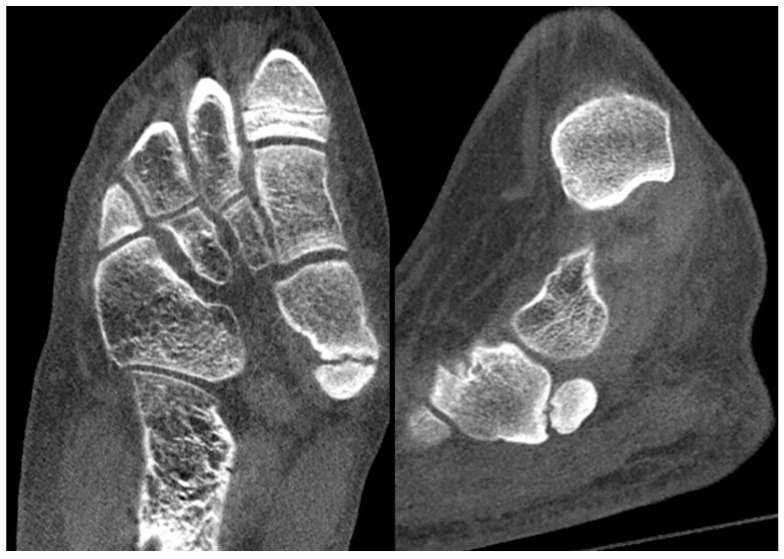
CT scan of a 12-year-old male patient showing a well-ossified accessory bone adjacent to the proximal medial margin of the navicular.
